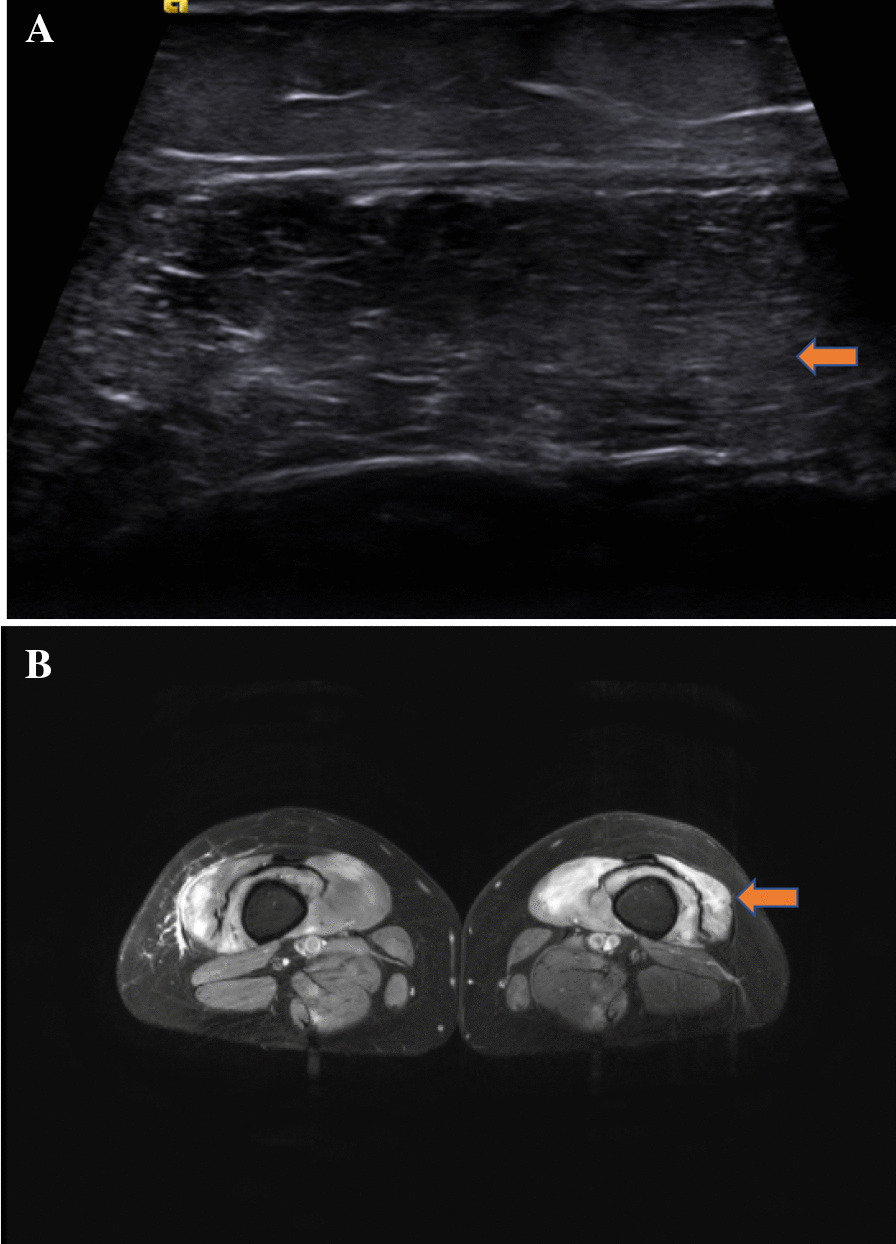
A US image showing patchy mild changes in echogenicity with hypoechoic areas and corresponding subcutaneous echogenicity in the vastus lateralis (cross-section) in a 26-year-old with Anti-NXP2. B The corresponding MRI in the same patient showed T2 (fat suppression) signal uptake indicative of muscle oedema in the vastus lateralis (arrowheads)
